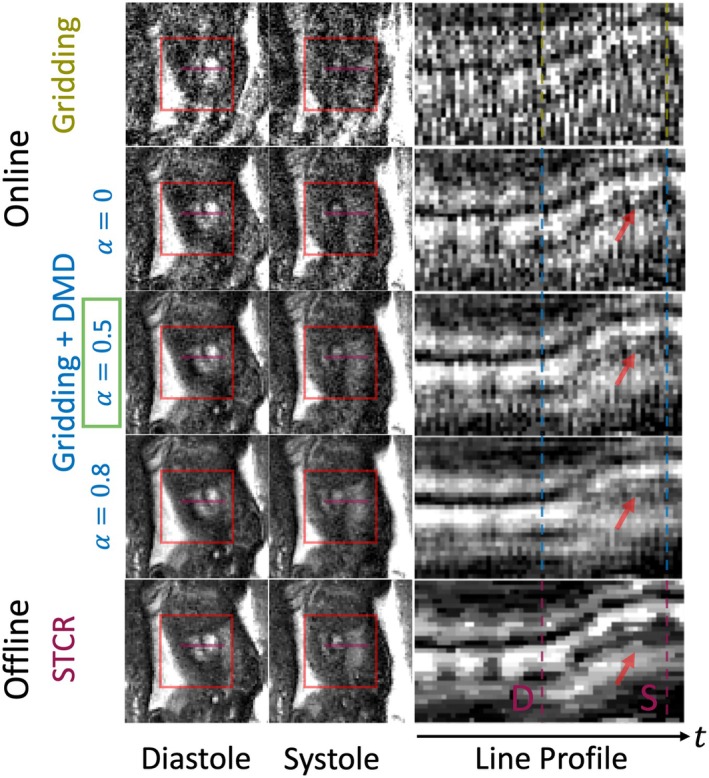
Impact of residue scale factor (α) on de‐aliasing performance for fetal cardiac RT‐MRI. The difference between the gridded input image series and the DMD model estimation, that is, residual signal, can be used to further suppress the aliasing. Specifically, the residual signal is scaled with parameter α∈[0,1] (residue scale factor) and added to the estimated aliasing with little computational overhead (no iterations). The effect of α is shown here for 0, 0.5 and 0.8 on an example fetal dataset with significant bulk motion (marked in the line profile), Video S3 shows the corresponding movie. Larger α values support better dealiasing performance at the cost of temporal smoothing, while smaller α values preserve the dynamics better at the cost of increased aliasing. We selected α=0.5 after a qualitative analysis on the fetal dataset as it provides a balance between the de‐aliasing performance and preservation of the dynamics.
